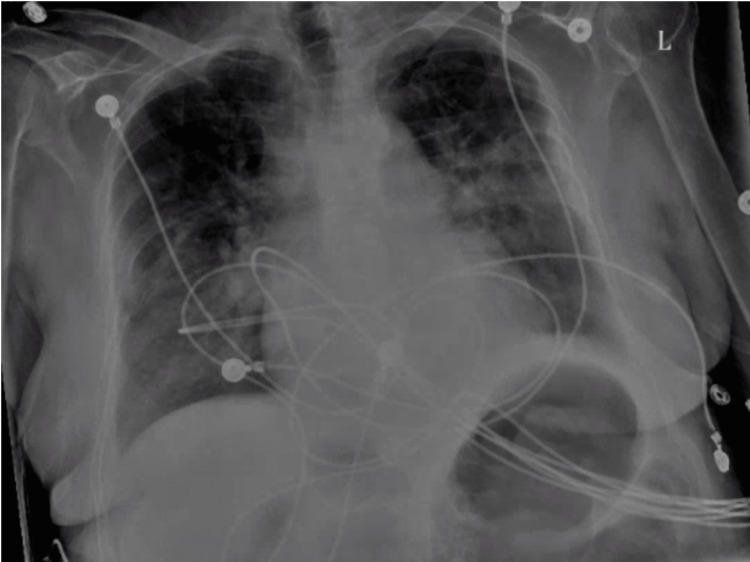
Chest X-ray on admission showing cardiomegaly with bilateral pulmonary edema Imaging obtained from the patient's chart
